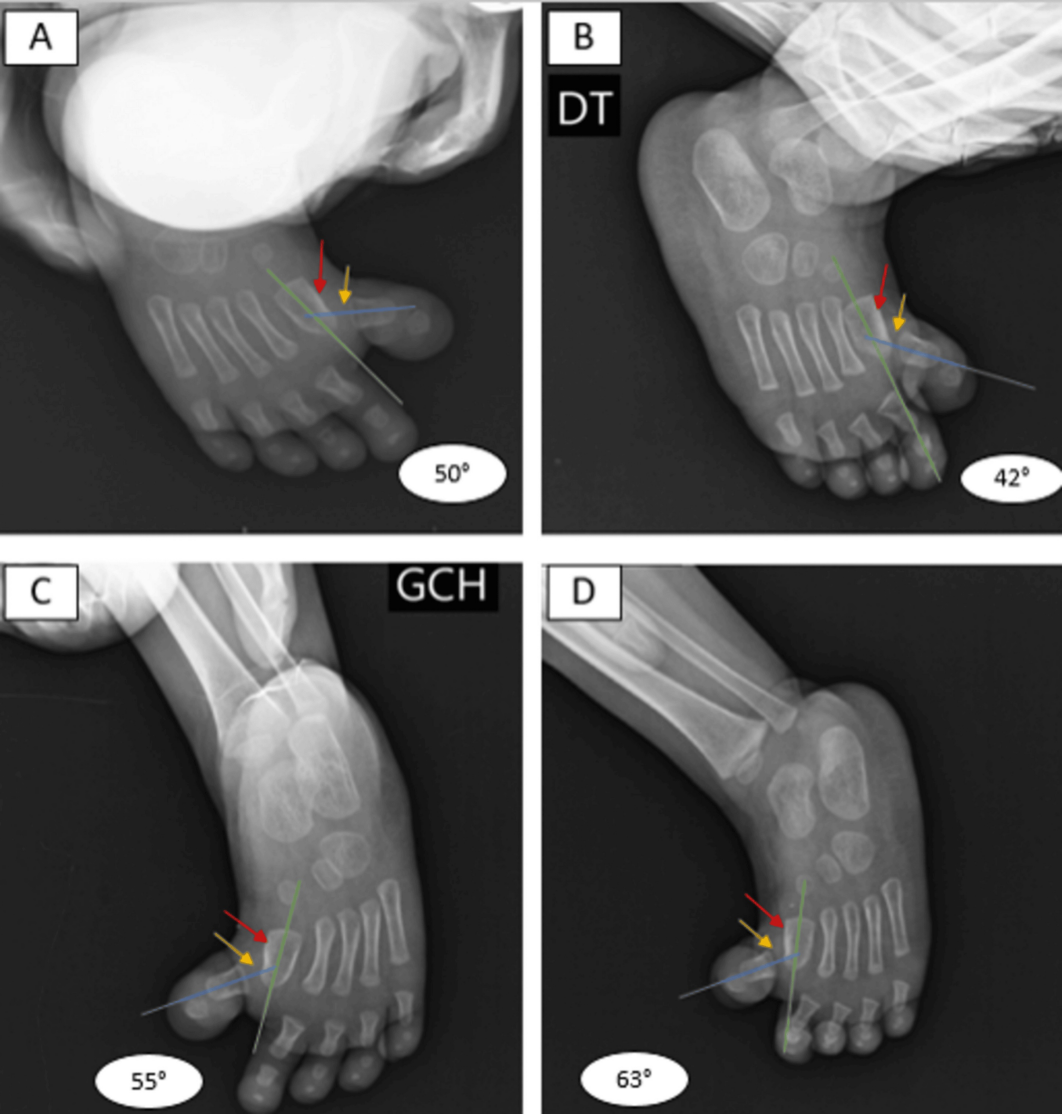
X-ray images of the patient Anteroposterior (A, C) and lateral (B, D) X-ray views of the right (A, B) and left (C, D) feet demonstrating bilateral congenital hallux varus. The deformities were localized at the first metatarsophalangeal (MTP) joint (yellow arrows), with a visibly shortened first metatarsal (red arrows). The angles of deviation were measured as follows: Right foot: 50° in the anteroposterior view (A) and 42° in the lateral view (B). Left foot: 55° in the anteroposterior view (C) and 63° in the lateral view (D). These measurements underscore the severity of the deformity.
